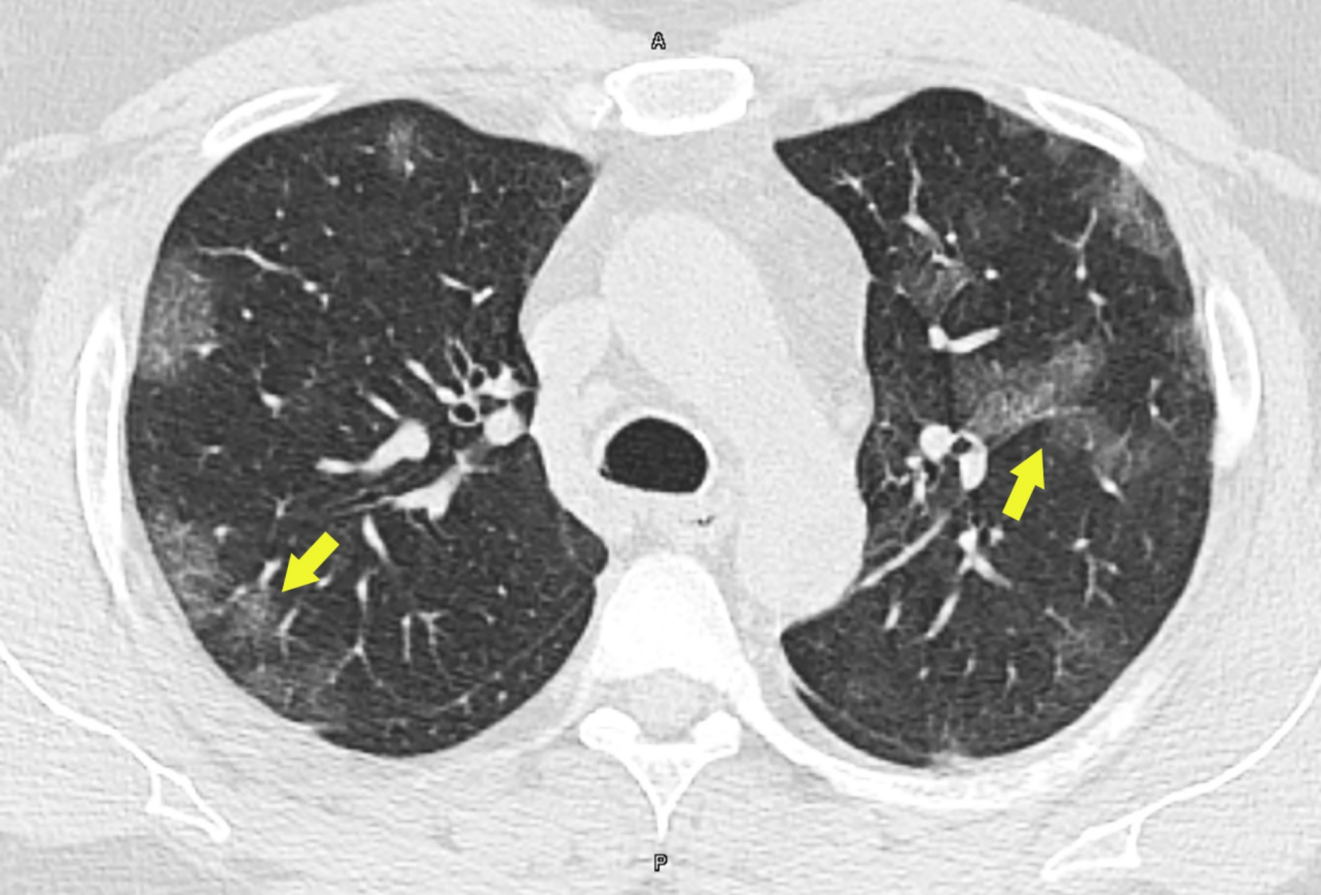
CT scan of thorax showing multifocal bilateral ground-glass lung opacities (arrows)
